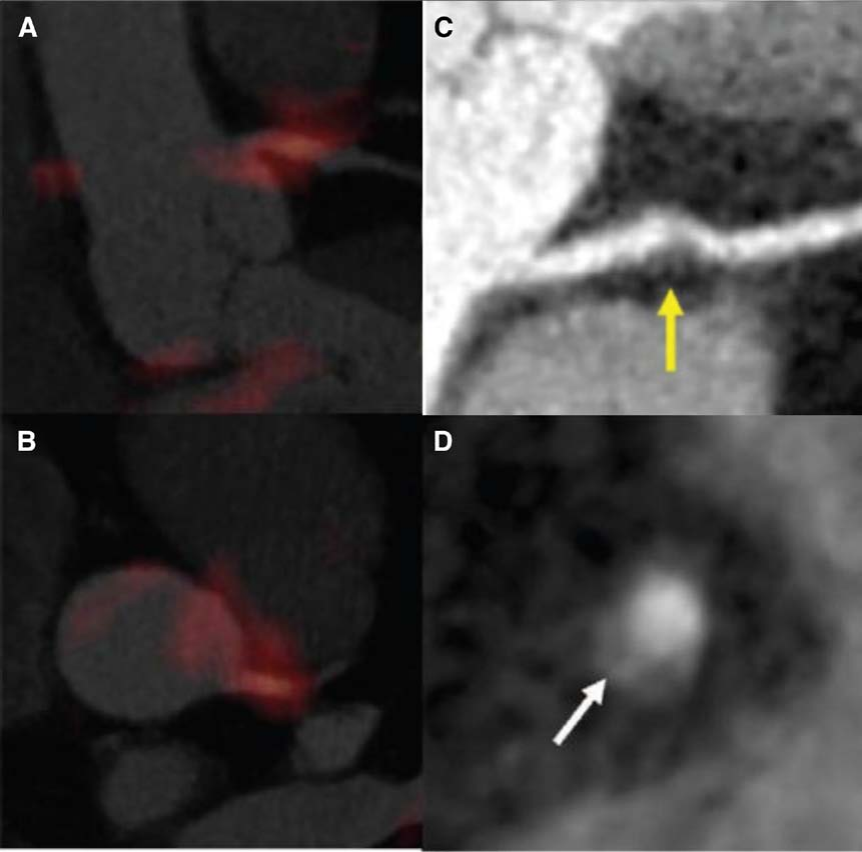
Focal ^18^F-flurodeoxyglucose (FDG) uptake in patients with high-risk plaque morphology in the left main coronary artery (LMCA). Fused positron emission tomographic (PET)/computed tomographic (CT) image showing intense and focal FDG uptake in the LMCA, in orthogonal images (A and B), corresponding maximum intensity projection–reconstructed computed tomography angiographic (CTA) image of LMCA with noncalcified plaque (arrow; C), and axial CTA showing a cross-sectional view (D) of an additional plaque in the right coronary artery manifesting positive remodeling and low attenuation (arrow) in the same subject.
